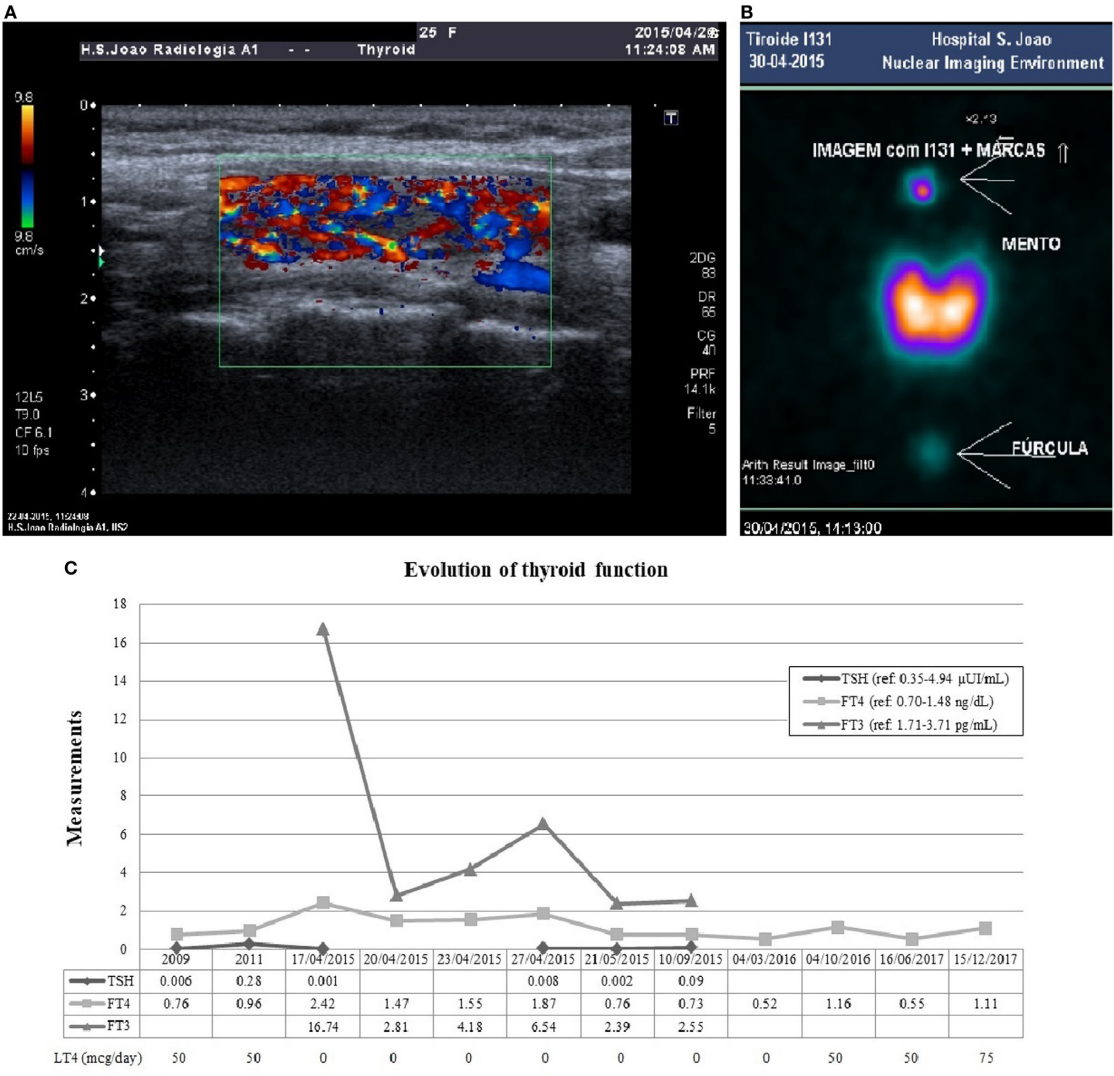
Thyroid ultrasonography (A) showed a normal size gland, with heterogeneous texture and pseudonodular areas, without nodular lesions, suggesting thyroiditis. The color flow Doppler signal showed significantly increased vascularity with diffuse homogeneous distribution (thyroid *inferno*). There was a markedly hyperfunctioning thyroid in scintigraphy (B), with homogeneous activity distribution and no focal areas suggestive of hyper- or hypoactive nodular formations. The radioactive iodine uptake was 70.2% at the end of 24 h, markedly elevated compared to normal range (10–30%). Panel (C) shows the evolution of thyroid function. After pituitary surgery in 2009 the patient developed secondary hypothyroidism and initiated LT4. She was admitted with primary hyperthyroidism in April 2015 and initiated MMI. During antithyroid drug withdrawal before scintigraphy, FT4 and FT3 re-increased above the reference range. MMI was progressively reduced after 6 months of treatment, but after withdrawal in October 2015, central hypothyroidism recurred and she resumed LT4 since March 2016. Abbreviations: LT4, levothyroxine; MMI, methimazole; FT4, free thyroxine; FT3, free triiodothyronine.
